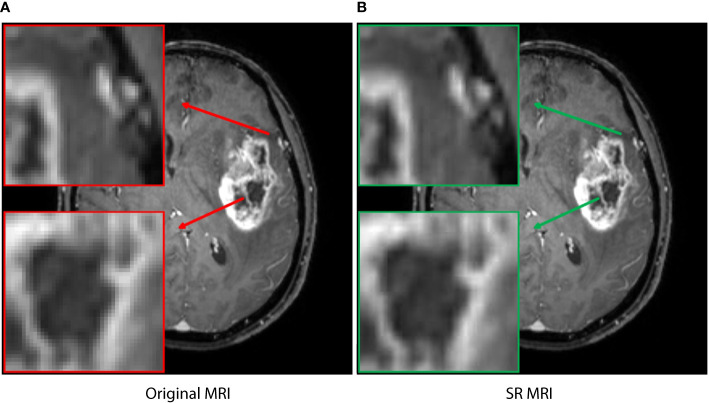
The super-resolution reconstruction result based on a generative adversarial network model. The reconstructed image (B) is not only very similar to the original transverse image (A), but also has more reasonable edges and finer textures. The boxes represent local enlarged images, with red and green arrows representing the original MRI and SR MRI, respectively.
